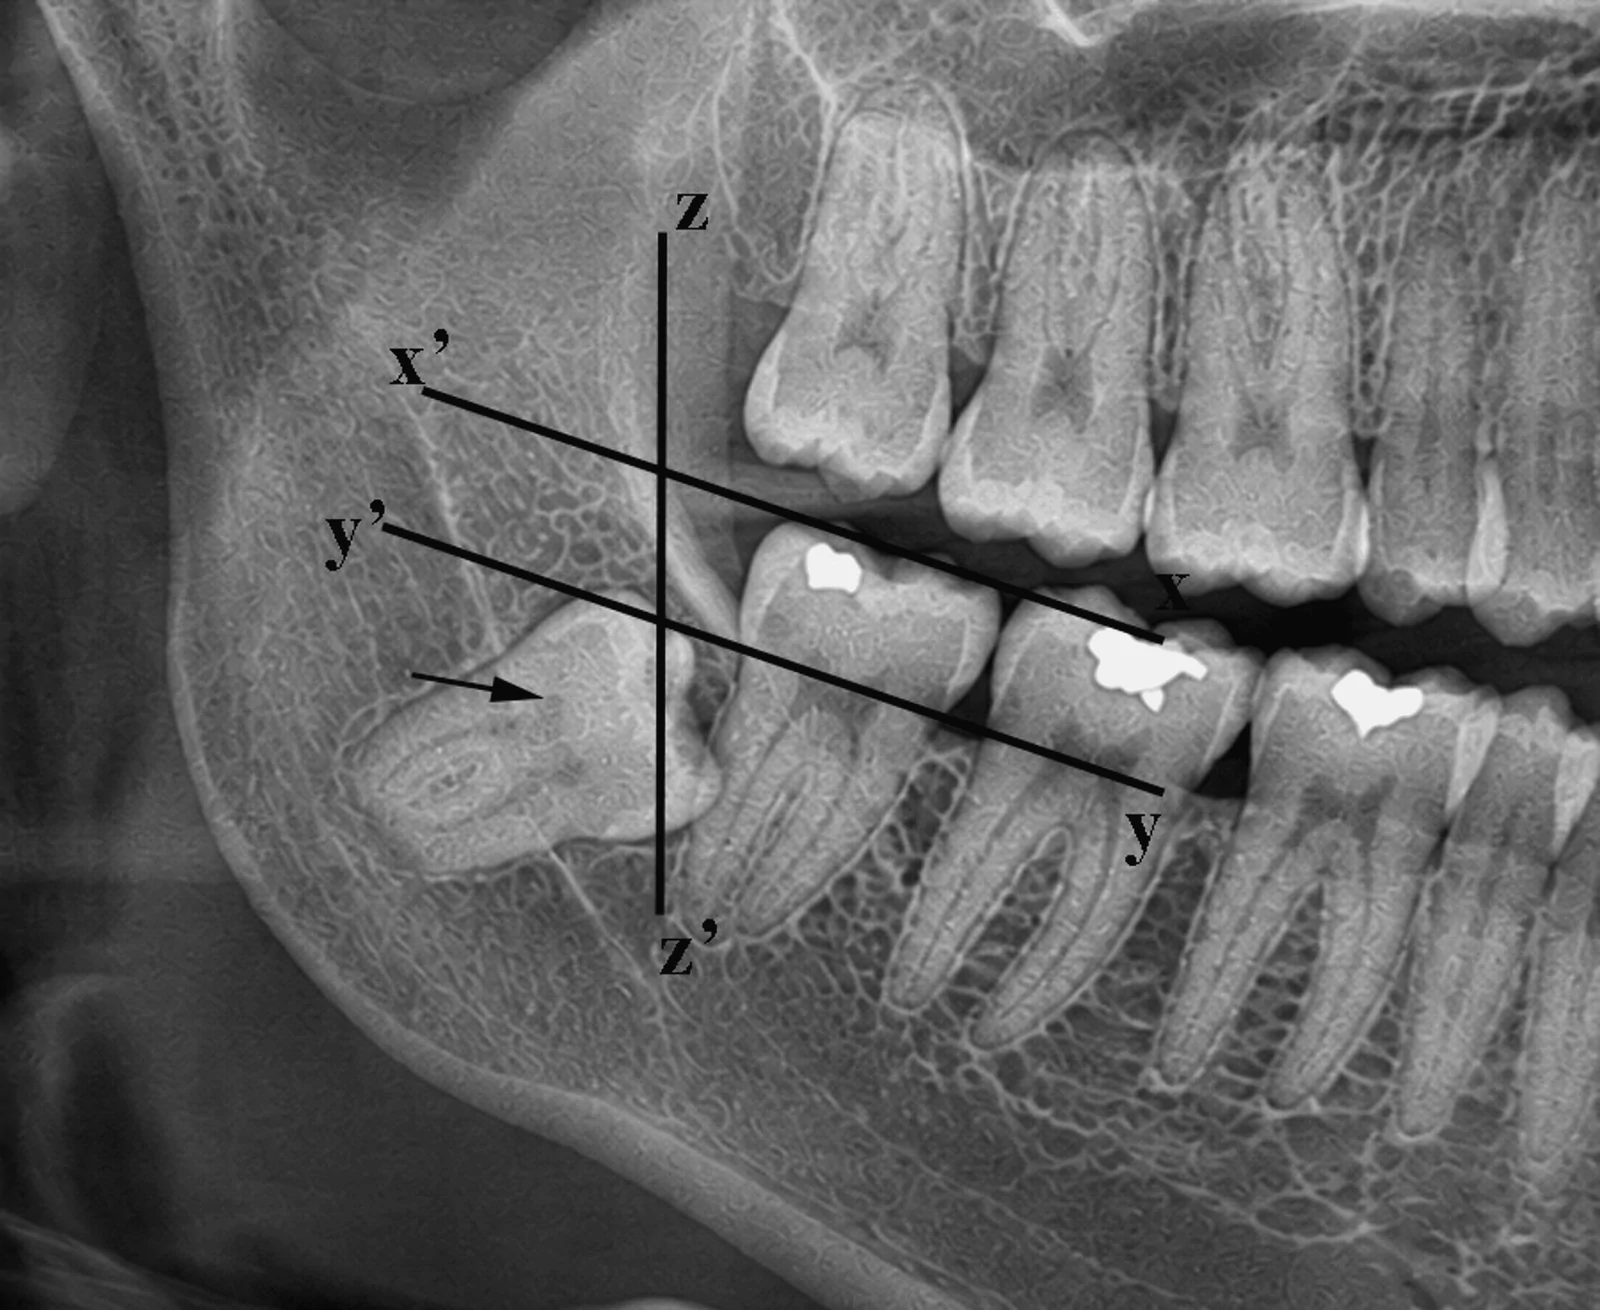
Representative panoramic radiograph demonstrating a mesioangular impacted mandibular third molar according to Winter's classification and classified as Pell and Gregory Level B and Class II. The x-x′ line represents the occlusal plane, the y-y′ line represents the reference line parallel to the occlusal plane used to assess the depth of impaction (Levels A-C), and the z-z′ line represents the anterior border of the mandibular ramus used to determine the ramus relationship (Classes I-III). The image is representative of the study sample.
